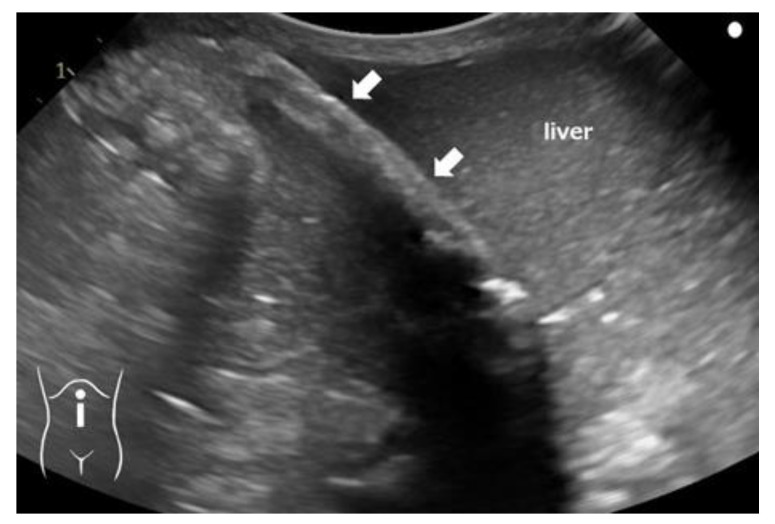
Longitudinal ultrasonographic image of the upper abdomen 11 days after birth. It shows an echogenic linear focus with posterior shadowing in the region of the umbilical vein (arrows).
